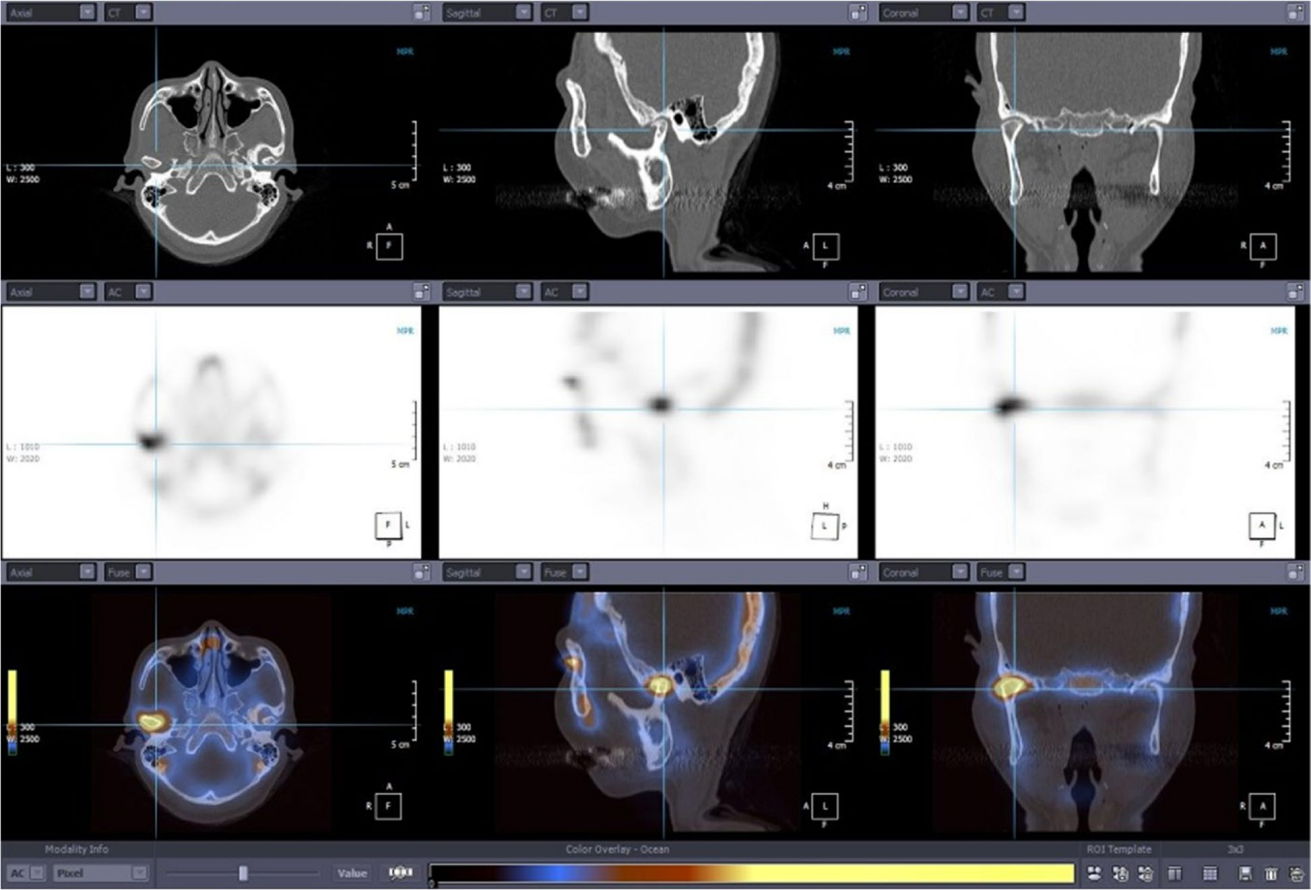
Facial CT, Bone SPECT and co-registered fused image using Xelis program in the transverse, coronal, and sagittal planes, showing focal hyperactivity of the radiopharmaceutical in the left TMJ
